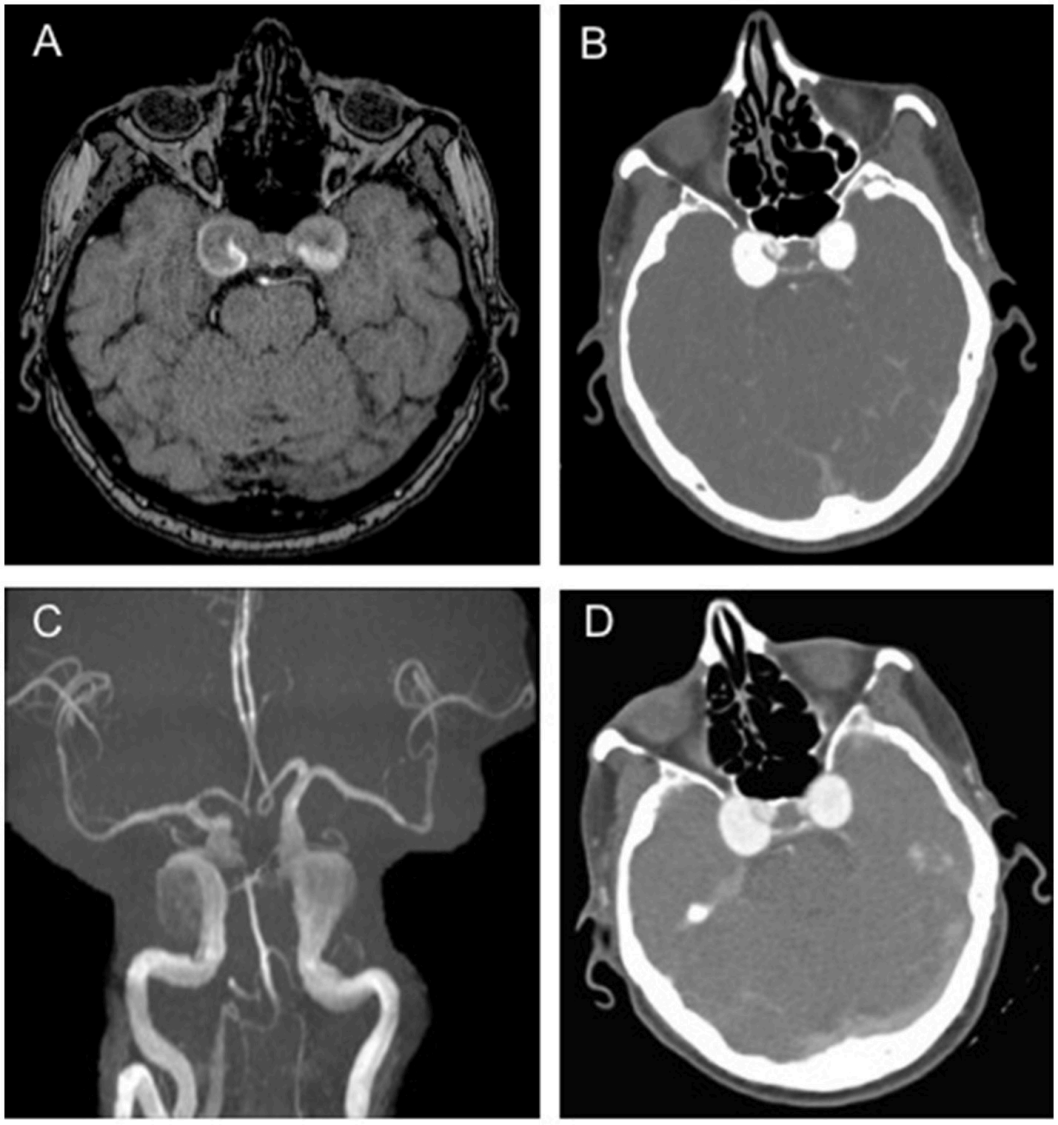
Brain imaging showing bilateral aneurysms.(A) T1-weighted axial brain magnetic resonance (MR) scan showing large bilateral cavernous carotid aneurysms (CCAs), with a larger multilobulated aneurysmal sac on the right; (B) Brain computed tomography (CT) angiography showing large bilateral CAAs measuring approximately 21 × 17 × 16 mm on the right and 18 × 15 × 16 mm on the left; (C,D) MR and CT angiography confirming the presence of large bilateral CAAs, unchanged in size from previous scans.
